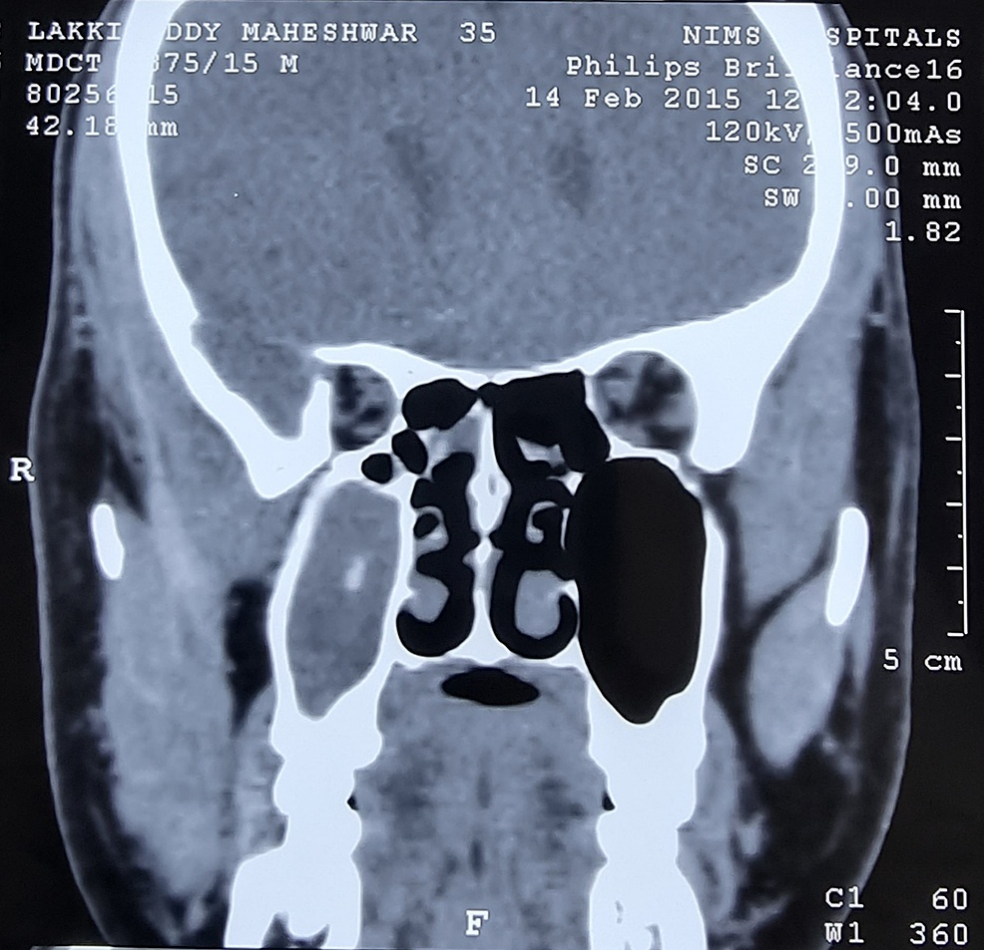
Coronal CT image of paranasal sinuses done in 2015 showing postoperative status with pansinusitis and significant mucosal thickening of the right maxillary sinus and double density sign signifying fungal sinusitis in the right maxillary sinus. CT: computed tomography
